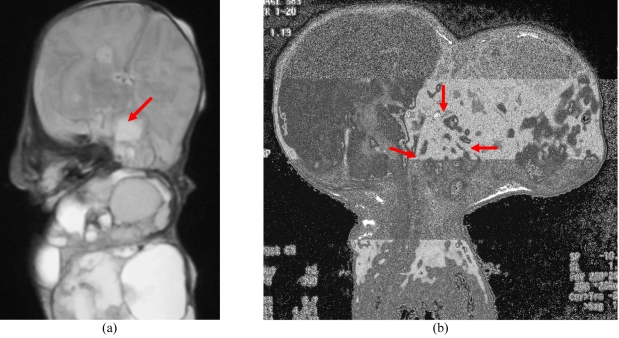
MR images: (a) T2 weighted ( TR 4500, TE 94) sequence in coronal plane showing the external portion of the tumour consisting of lobulated well defined areas of fluid, solid tissue and fat, in keeping with teratoma. There is involvement of the left temporal lobe of the brain (red arrow); (b) T1 weighted (TR 9.7, TE 4.1) contrast enhanced sequence in sagittal plane. Internally, the tumour is occupying the oropharynx, nasopharynx and oral cavity, effacing normal anatomy (red arrows).
